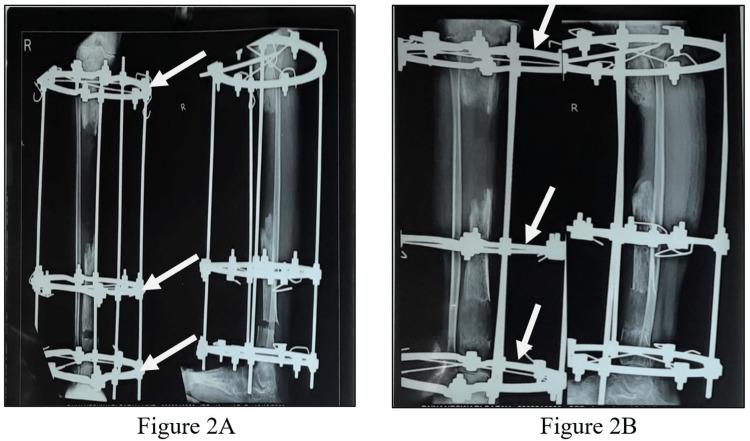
Check X-rays of the right lower leg with Ilizarov ring fixator in situ: A) knee AP view, B) lower leg AP and lateral view anteroposterior: AP
